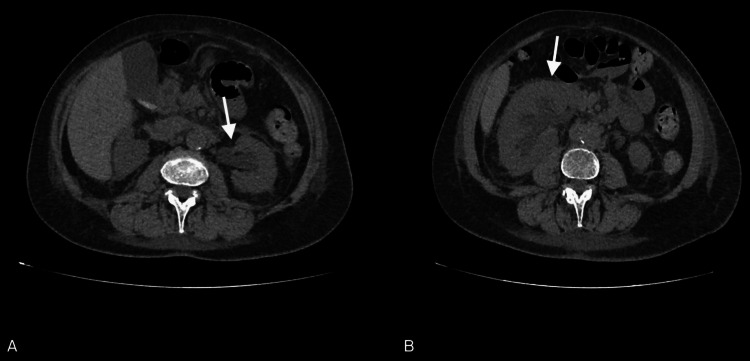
CT abdomen: left caliceal, pelvic, and ureteral dilatation (arrow in A) and right kidney medialized and swollen (arrow in B). There is modest dilatation of the calyceal cavities and the renal pelvis, as well as of the proximal tract of the ureter in the absence of definite images suggestive of lithiasis. Minimal thickening of the perirenal fascia is also associated (A). Diffuse heterogeneity of mesenteric adipose tissue, right kidney slightly medialized, increased in size with a swollen appearance, modest dilatation of the calyceal cavities and the right renal pelvis as well as of the proximal tract of the ureter. Thickening of the perirenal fascia is associated with heterogeneity of the anterior and posterior pararenal spaces (B).
